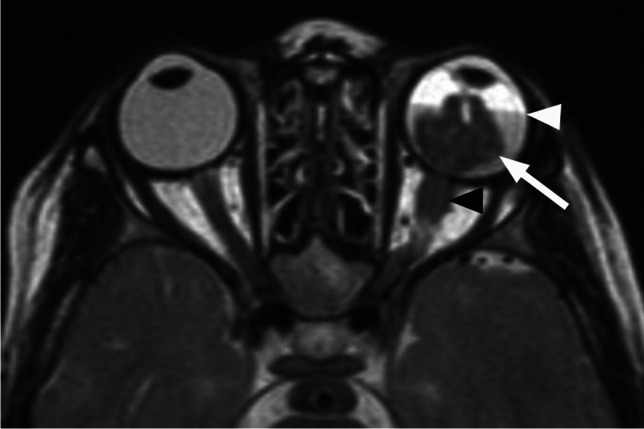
Retinoblastoma with postlaminar optic nerve invasion (PLONI). Axial T2-weighted image shows left intraocular hypointense mass (arrow) with retinal detachment and subretinal fluid level (white arrowhead). There is also thickening of the retrobulbar optic nerve (black arrowhead)
